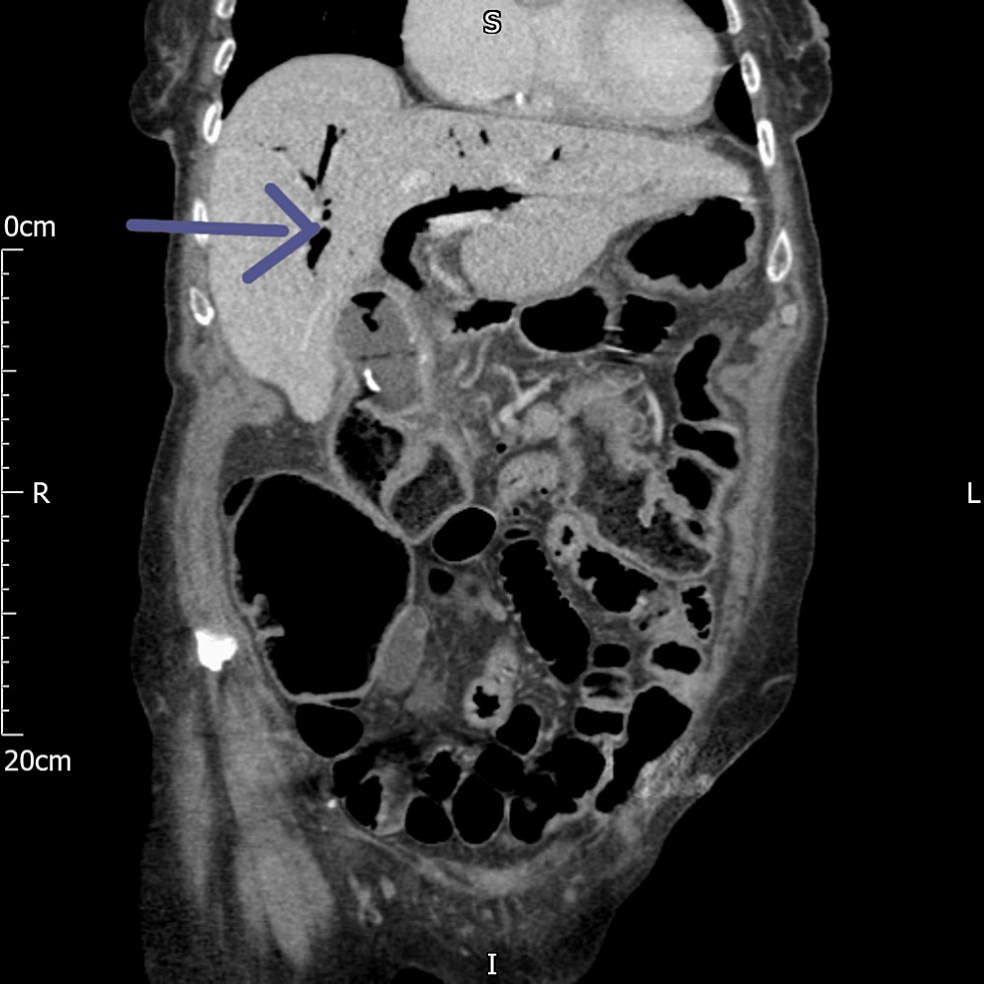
CT of the abdomen coronal view showing extensive pneumobilia (the blue arrow) CT: computed tomography
